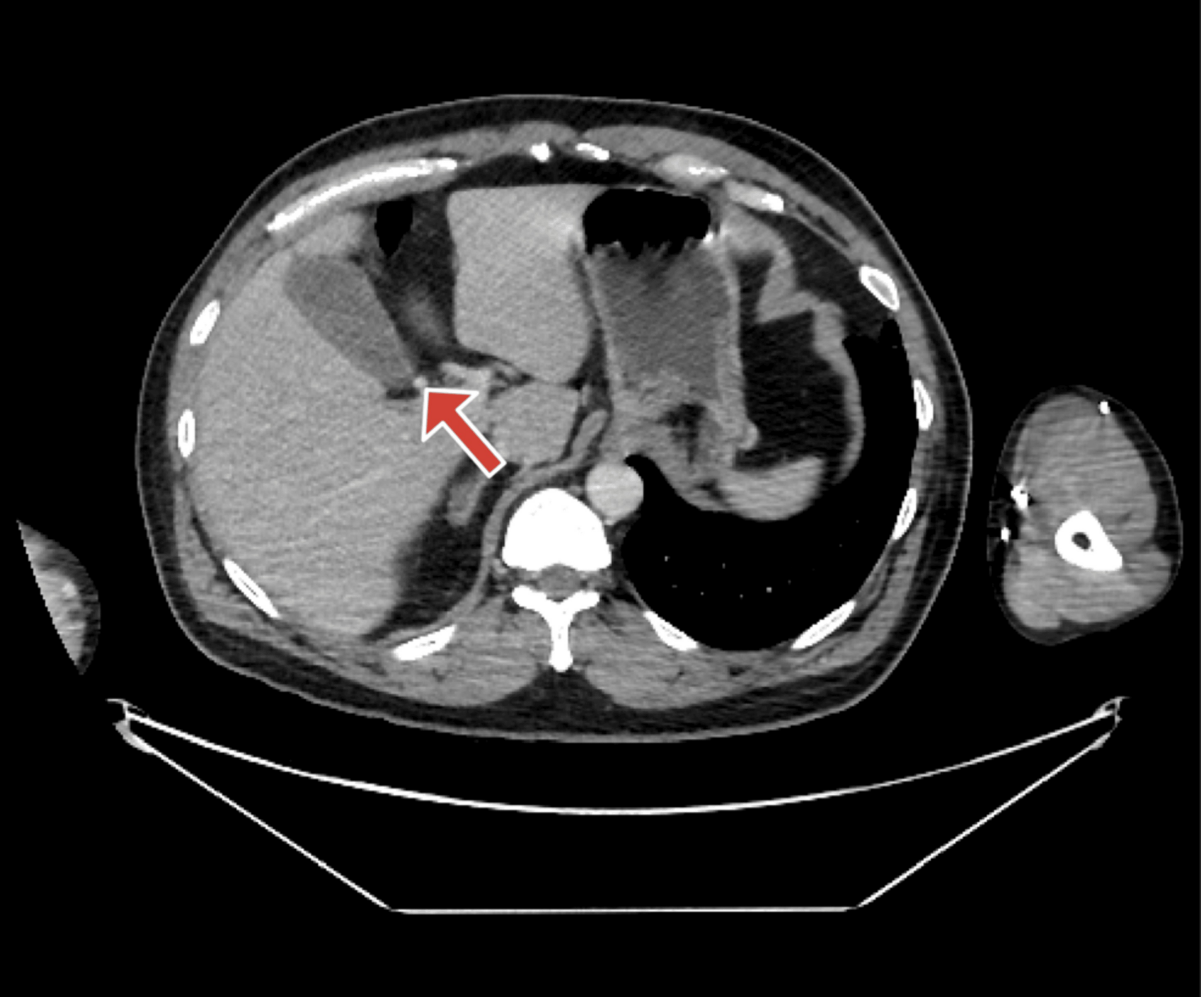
Computed tomography (CT) image of the right hepatic artery arching behind the common bile duct (CBD) Contrast-enhanced abdominal CT, axial view in the portal venous phase, obtained at a more superior level than Figure 2, demonstrating the right hepatic artery coursing posterior to the common bile duct and continuing distally (arrow).
